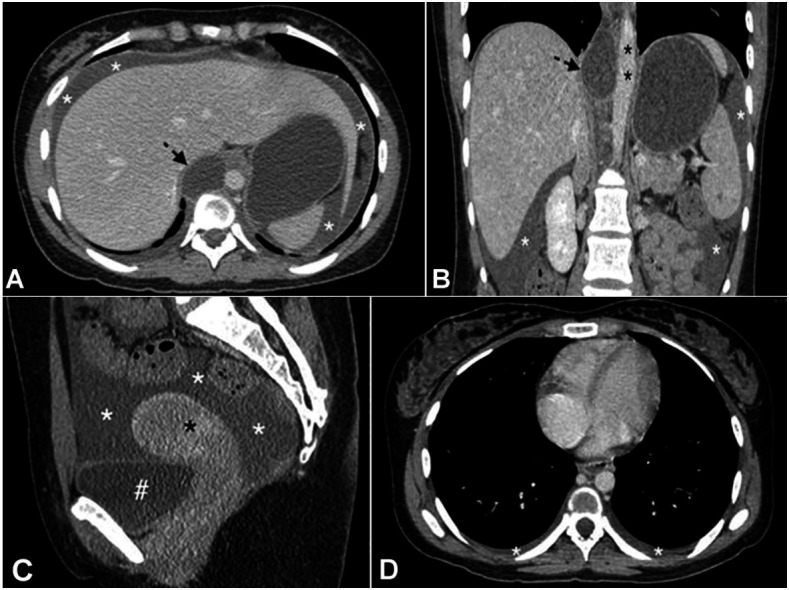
Thoracic and abdominal enhanced computed tomography. A – axial plane and B coronal plane – images at admission showing a cystic image (black arrow) in the thoracic duct site at the thoracic-abdominal transition, well-defined and without enhancement in contact with the aorta (black asterisk), and associated with ascites (white asterisks), C – sagittal plane and D axial plane showing ascites (white asterisks) and bilateral laminar pleural effusion, as well as the bladder (#) and the uterus (black asterisk).
